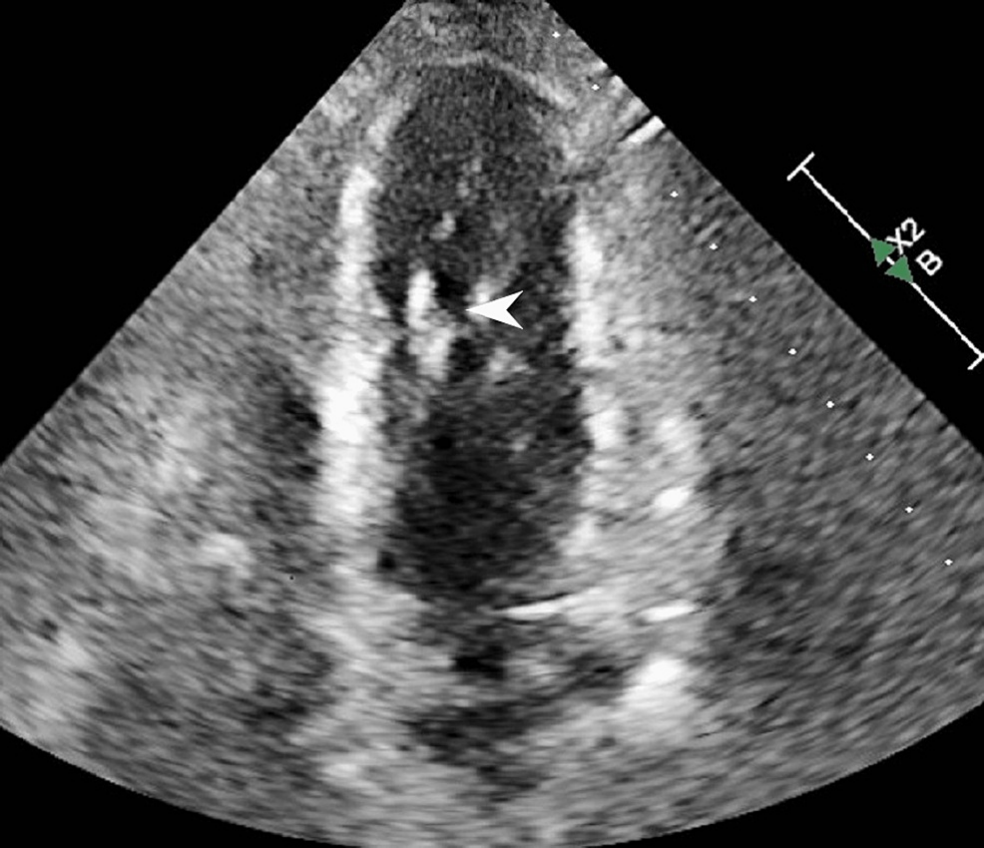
2D echocardiogram of the left ventricle in systole. Note the visualized close approximation of the endocardial border (arrow). 2D: Two-dimensional
